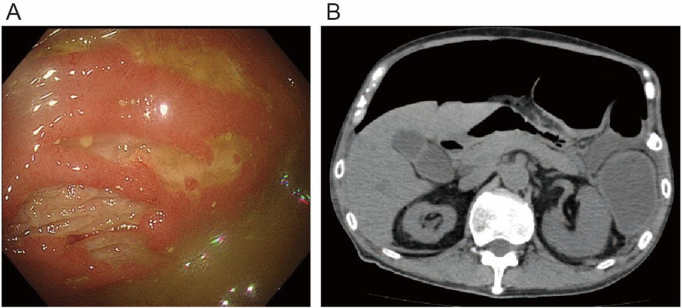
Colonoscopy and computed tomography findings. (A) Colonoscopy showing ulcerating lesions in the descending colon. (B) Abdominal computed tomography showing free air and ascites.
